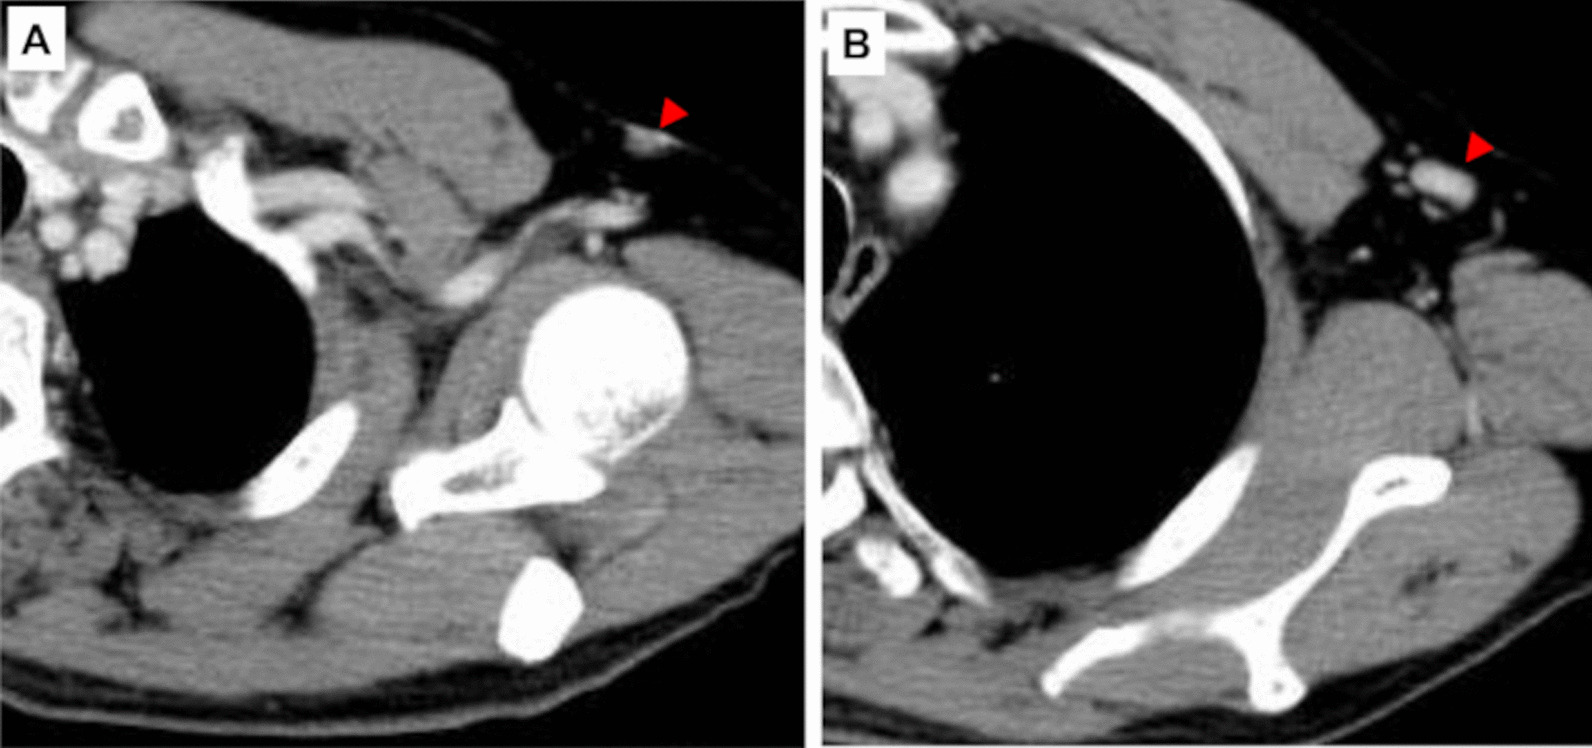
Computed tomography (CT). A CT shows an irregular mass in the left axilla (red arrowhead). B Some enlarged axillary lymph nodes are seen (red arrowhead)
